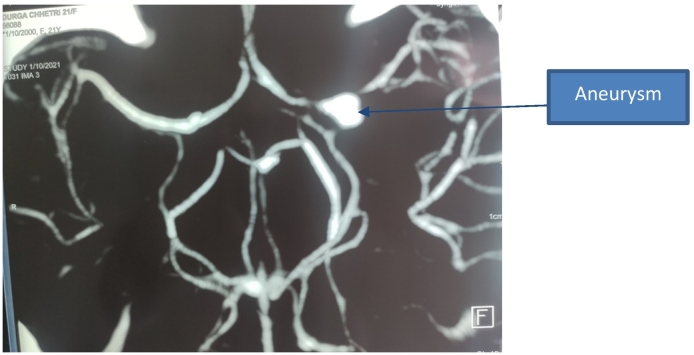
CT Angiogram shows left ICA bifurcation aneurysm measuring directed superolateral aneurysm Left pterional craniotomy and microsurgical clipping of aneurysm were performed. Intraoperative findings showed a bilobed saccular aneurysm, arising from left internal carotid artery bifurcation. The Postoperative was uneventful and the patient was discharged without deficits.
